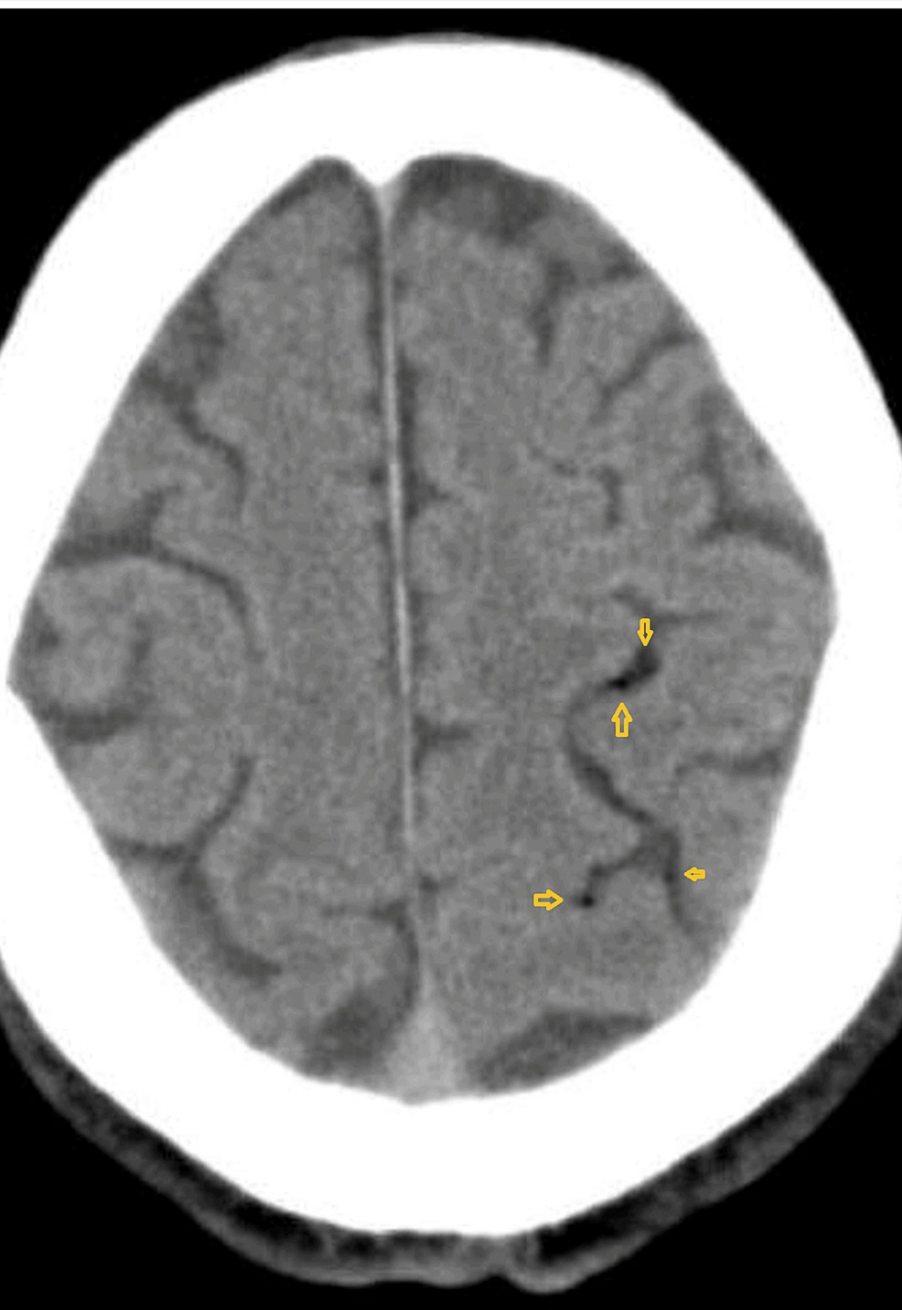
Axial Brain CT scan which revealed small low-density spots at the left parieto-occipital region suggestive of subarachnoid air bubbles (arrows) due to air embolism
